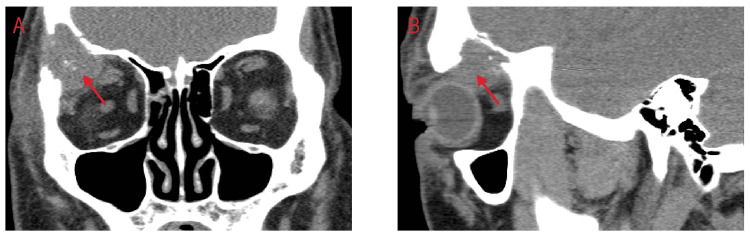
CT imaging of the orbits. (A) Coronal and (B) sagittal views showing a lytic lesion with a soft tissue mass in the lateral wall and roof of the right orbit with a mass effect on the superior and lateral rectus muscles and on the right globe.
